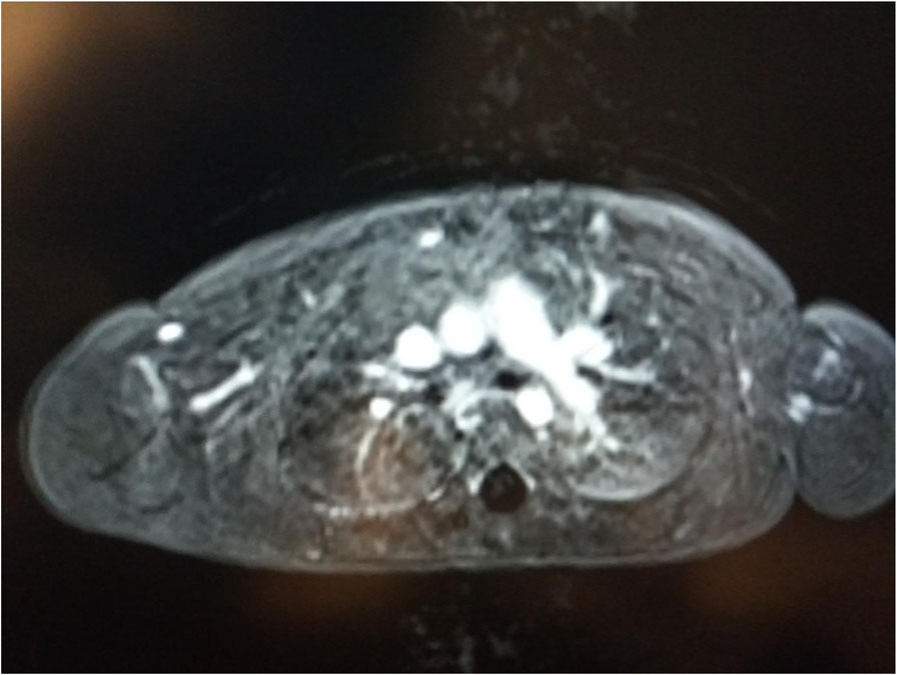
Chest MRI, at one month of age, showing isolated unilateral absence of the right pulmonary artery
